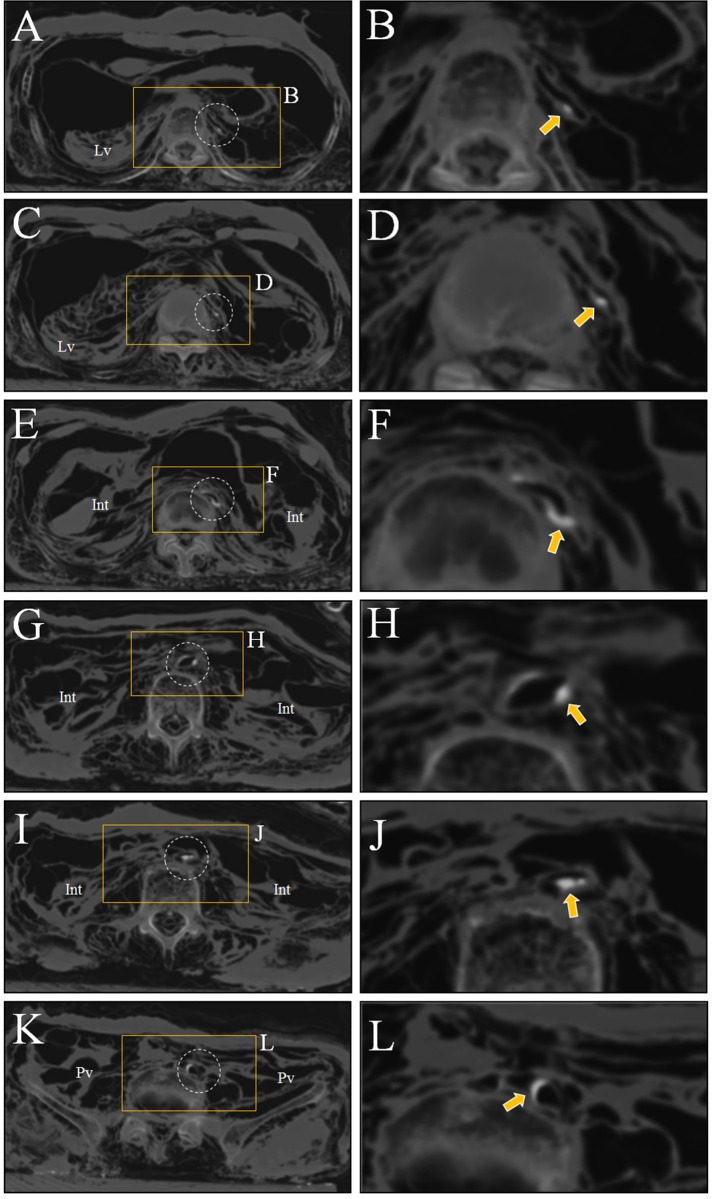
The CT images showing calcifications in aorta wall. (A) to (D) Upper abdomen. Calcifications (pointed by yellow arrows) are seen in the wall of abdominal aorta (dotted circles). (B) and (D) are magnified images of (A) and (C), respectively. Lv, liver. (E) to (H) The axial view through umbilicus. Calcifications are still identified in aorta walls. Int, intestines. (F) and (H) are magnified image of (E) and (G), respectively. (I) to (L) The axial view of pelvic region. Aortic calcification could be also observed. (J) and (L) are magnified images of (I) and (K), respectively. Pv, pelvis.
